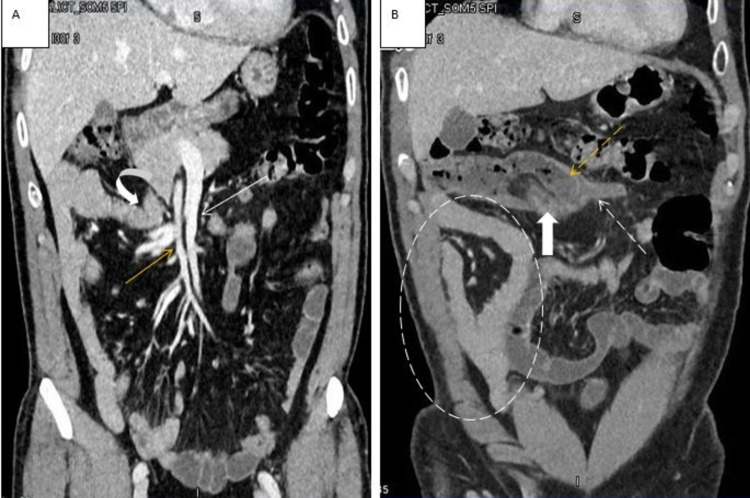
Coronal reformation of contrast-enhanced CT abdomen. Contrast-enhanced CT abdomen showing SMA (orange arrow in A) to the right of SMV (white arrow in A), duodenojejunal junction to the right of the midline (curved arrow in A), small bowel jejunal loops on the right side (dashed circle in B), and the cecum in the midline supraumbilical position (dashed orange arrow in B) with the arising inflamed appendix (dashed white arrow in B). The ileocecal valve with the surrounding fat is also demonstrated (block arrow in B). CT: computed tomography; SMA: superior mesenteric artery; SMV: superior mesenteric vein
